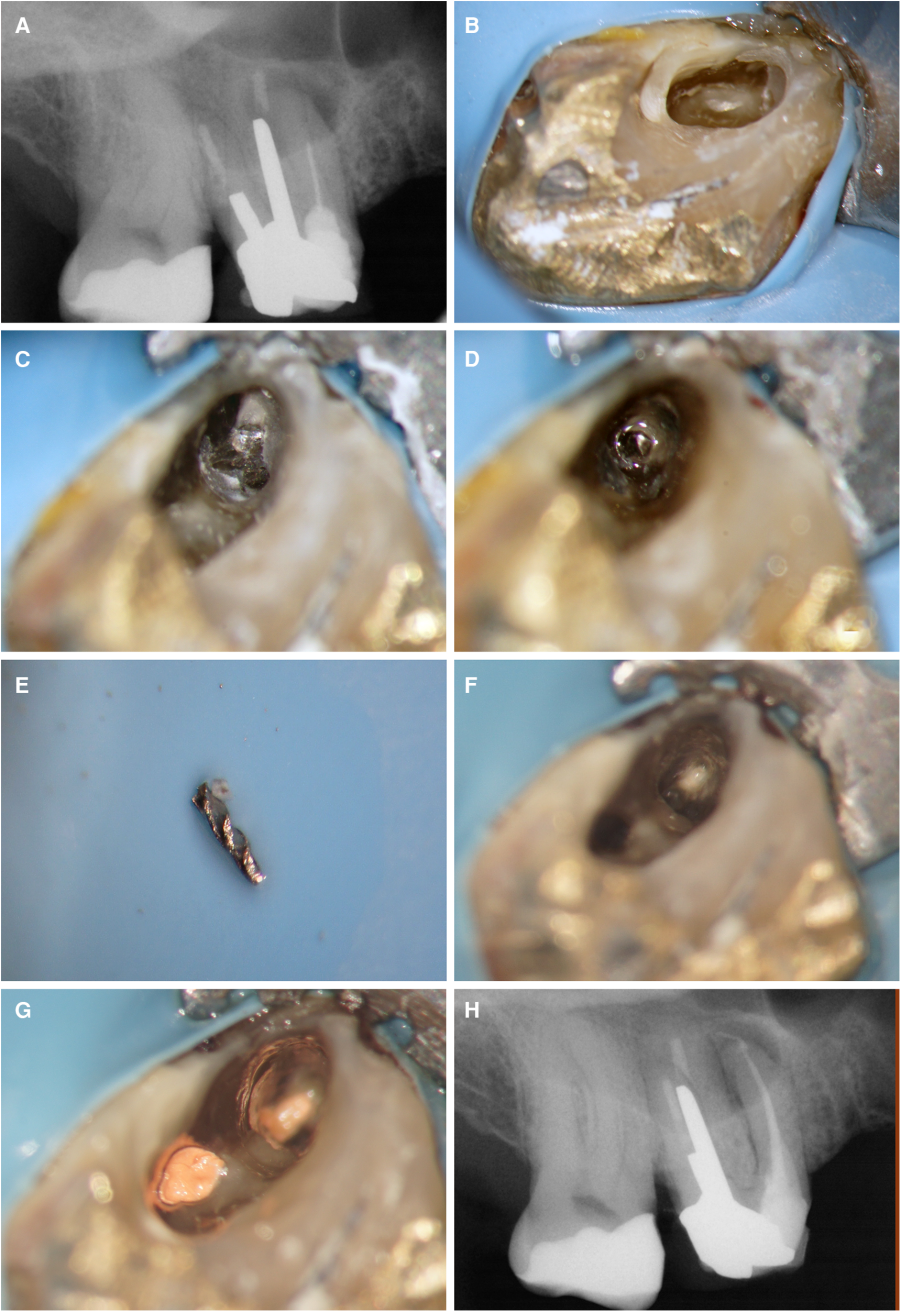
Overview of the endodontic procedure: (A) preoperative periapical radiograph, (B) access cavity preparation, (C) identification of the broken instrument within the canal, (D) creation of space surrounding the broken instrument, (E) successful removal of the broken instrument, (F) location and preparation of the MB2 canal, (G) root canal filling of both canals, and (H) postoperative periapical radiograph.
